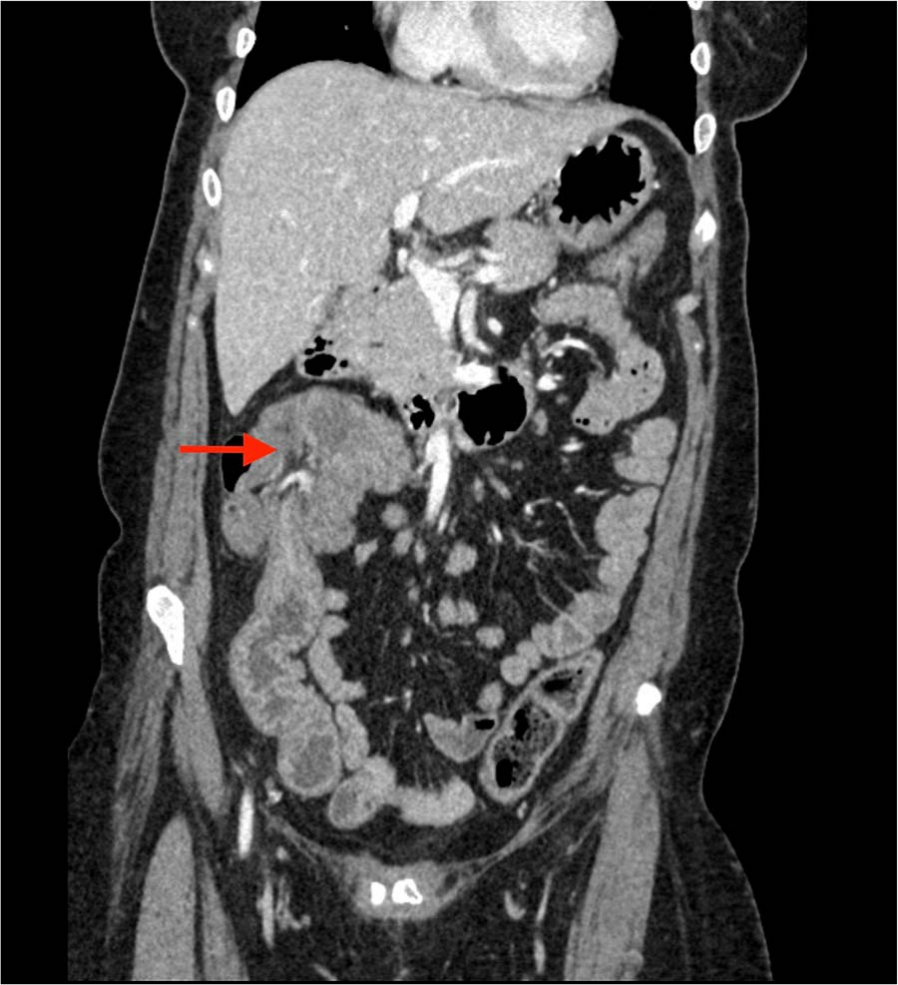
Contrast enhanced CT of the abdomen showing an arrow pointing to the region of ileocolic intussusception.
